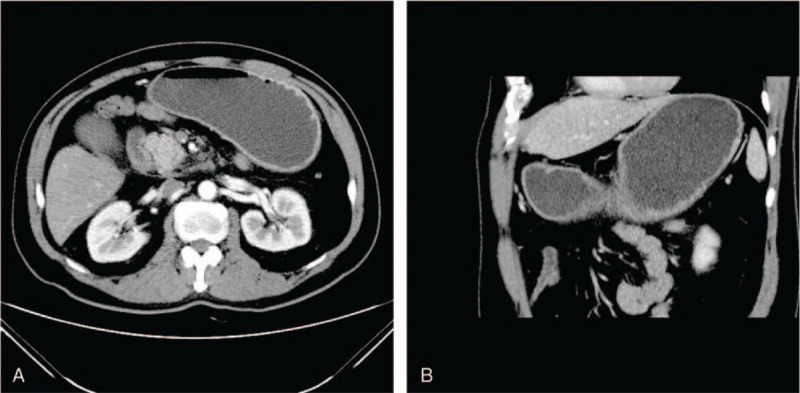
(A) Contrast-enhanced computed tomography showing improvement in swelling of the pancreatic head, Thickening of the duodenal wall. (B) Gastric outlet obstruction.
